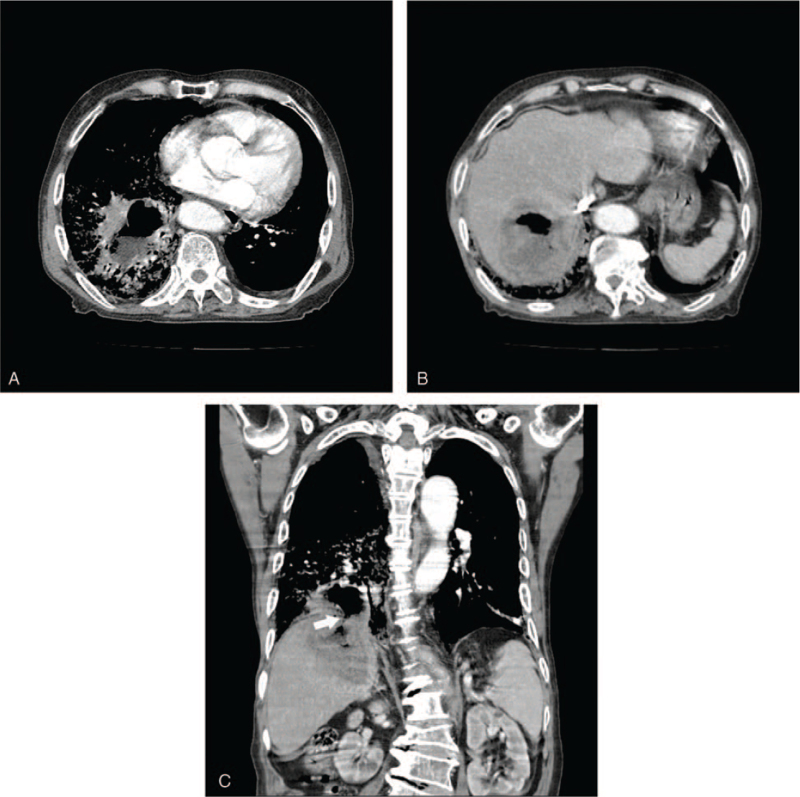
(A) A computed tomography (CT) scan of the chest depicts a 6.1-cm-sized air- and fluid-filled cavitary lesion with surrounding air space consolidation in the right lower lung field. (B) CT indicates a liver abscess with thick walls at segment 7 of the liver. (C) A fistula at the diaphragm between the 2 abscesses is visible in the coronal plane (arrow).
